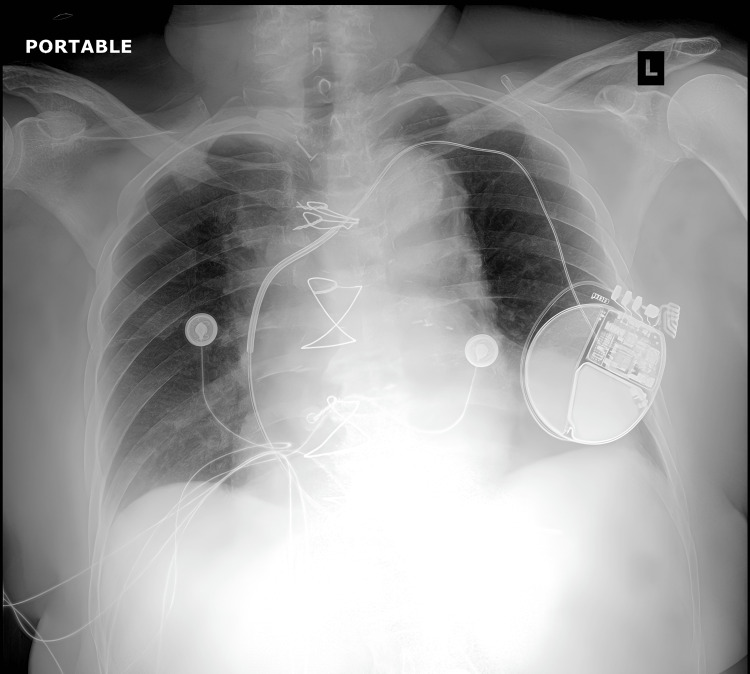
Chest X-ray failing to demonstrate overt left heart failure
